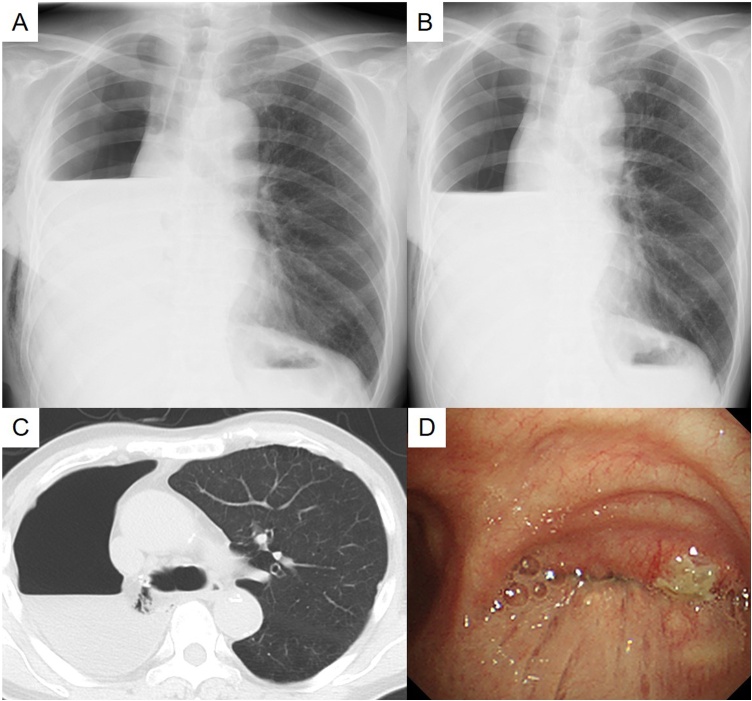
(A) Chest radiogram taken on the 4th postoperative day. (B) The air-fluid level of the right pleural cavity suddenly decreased on the 19th postoperative day. (C) The air was then detected around the bronchial stump using computed tomography. (D) Bronchoscopy was not able to detect the fistula of the bronchial stump.
